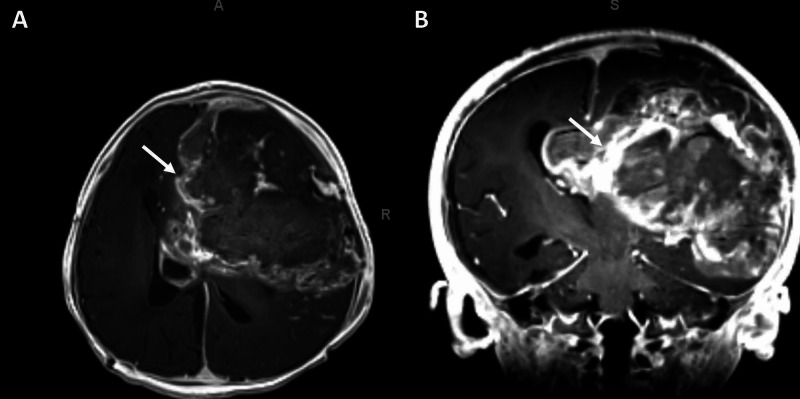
Post-resection imaging MRIs status post the first subtotal resection. (A) Axial MRI with residual peripheral areas of enhancement most predominantly in the left temporal lobe (arrow). (B) Coronal MRI showing intrinsic T1 hyperintense areas of hemorrhage within the surgical cavity (arrow)
